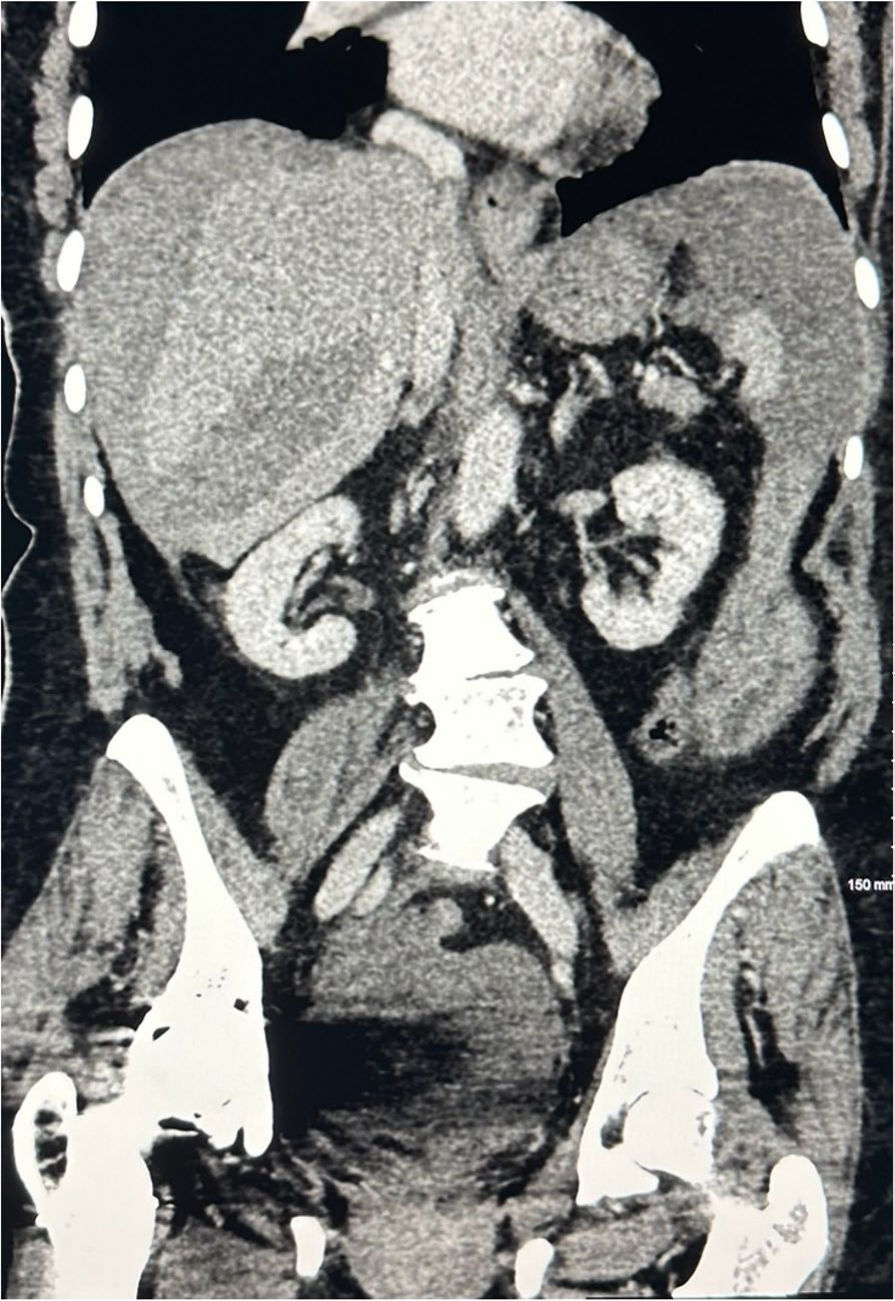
Coronal portal venous phase CT abdomen and pelvis showing hepatic laceration with ruptured capsule and subcapsular haematoma
